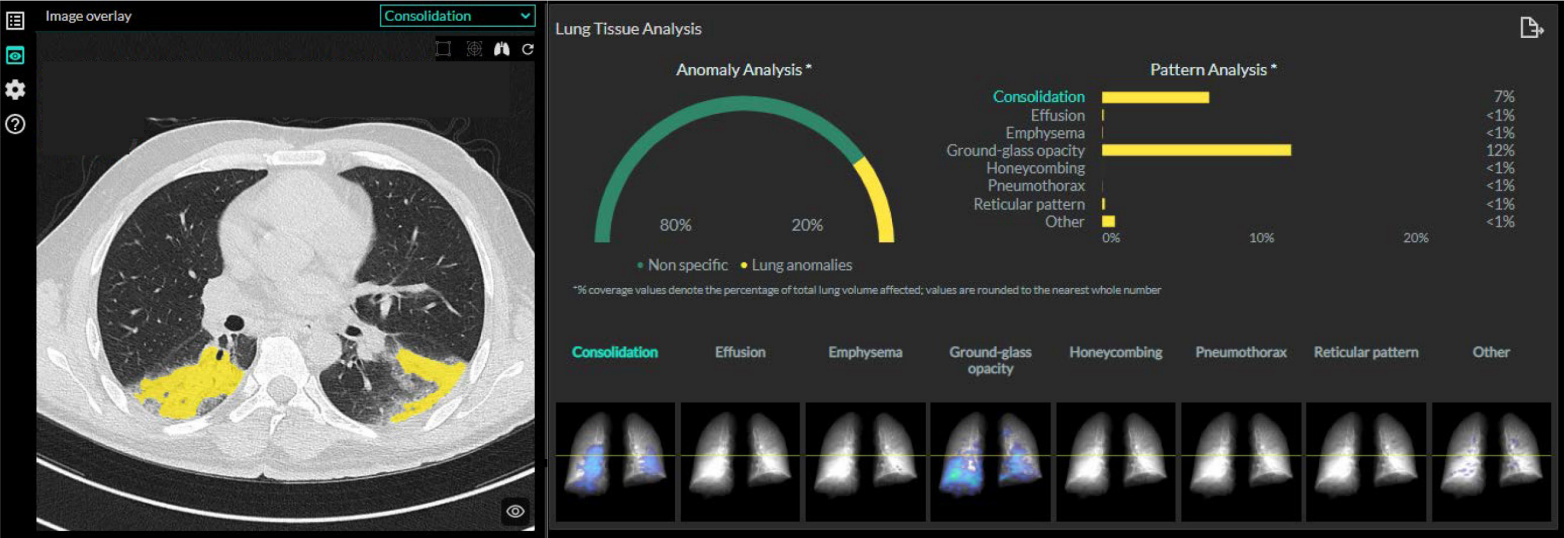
Example of a quantitative pattern analysis report regarding the percentage of lung anomalies (consolidation and ground-glass opacities) detected on a chest CT scan of a 42-year-old-patient with COVID-19 pneumonia.
